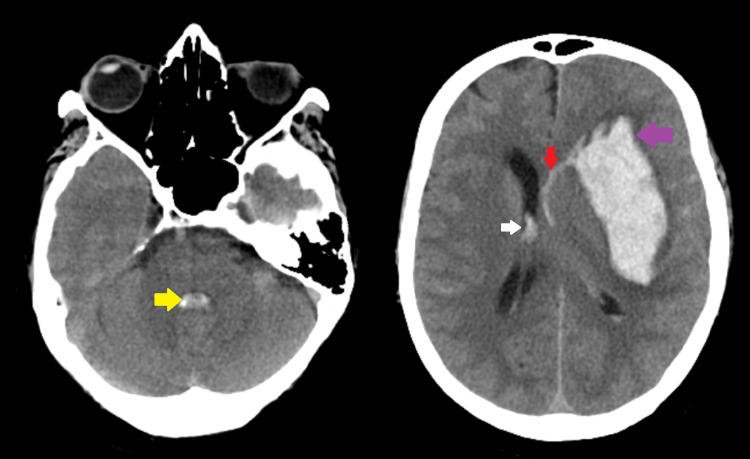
CT brain without contrast reveals subarachnoid hemorrhage (red arrow), intraparenchymal hemorrhage in the left frontotemporal lobe and lentiform nucleus (purple arrow), intraventricular extension (white arrow), and brainstem bleed (yellow arrow)
